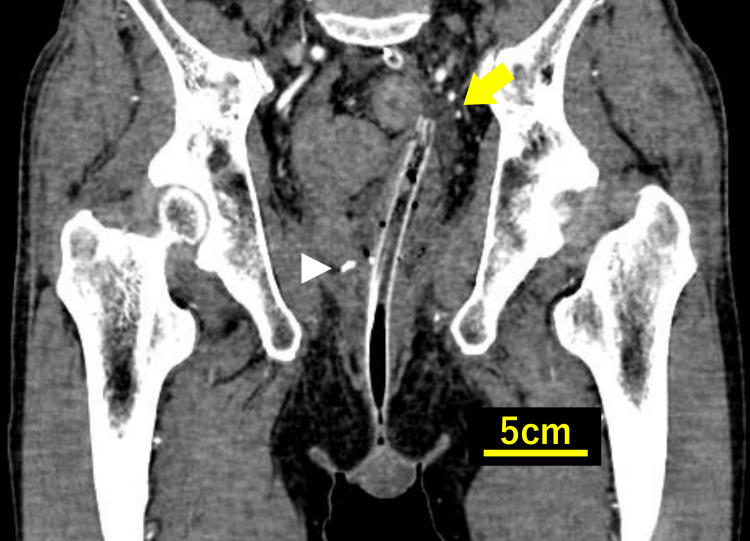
Contrast-enhanced CT image (coronal view) showing the transanal drainage tube (yellow arrow) deviating to the left and slightly penetrating the bowel wall. The anastomotic site is indicated by a white arrowhead. (CT slice thickness: 1 mm, portal venous phase).
